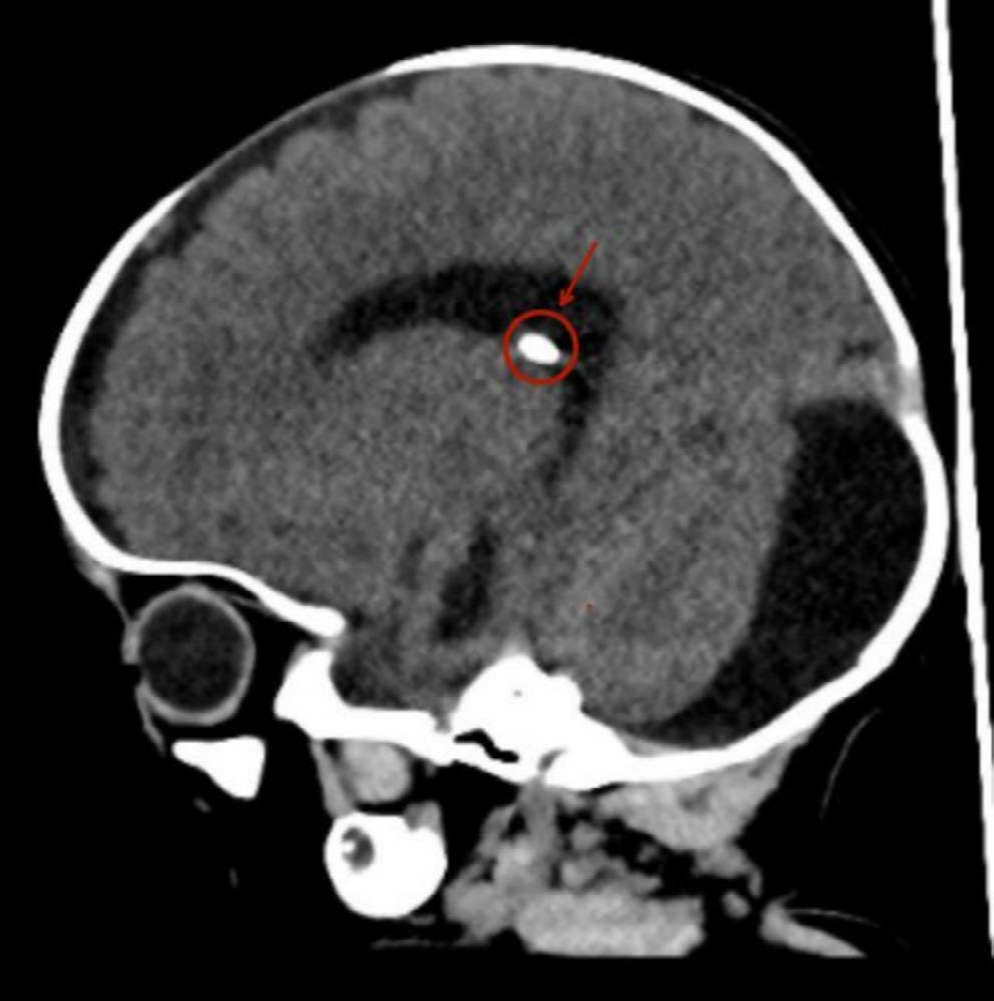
CT Scan of the brain. Ventricular drainage device, shown in red, seen within the lateral ventricle.
